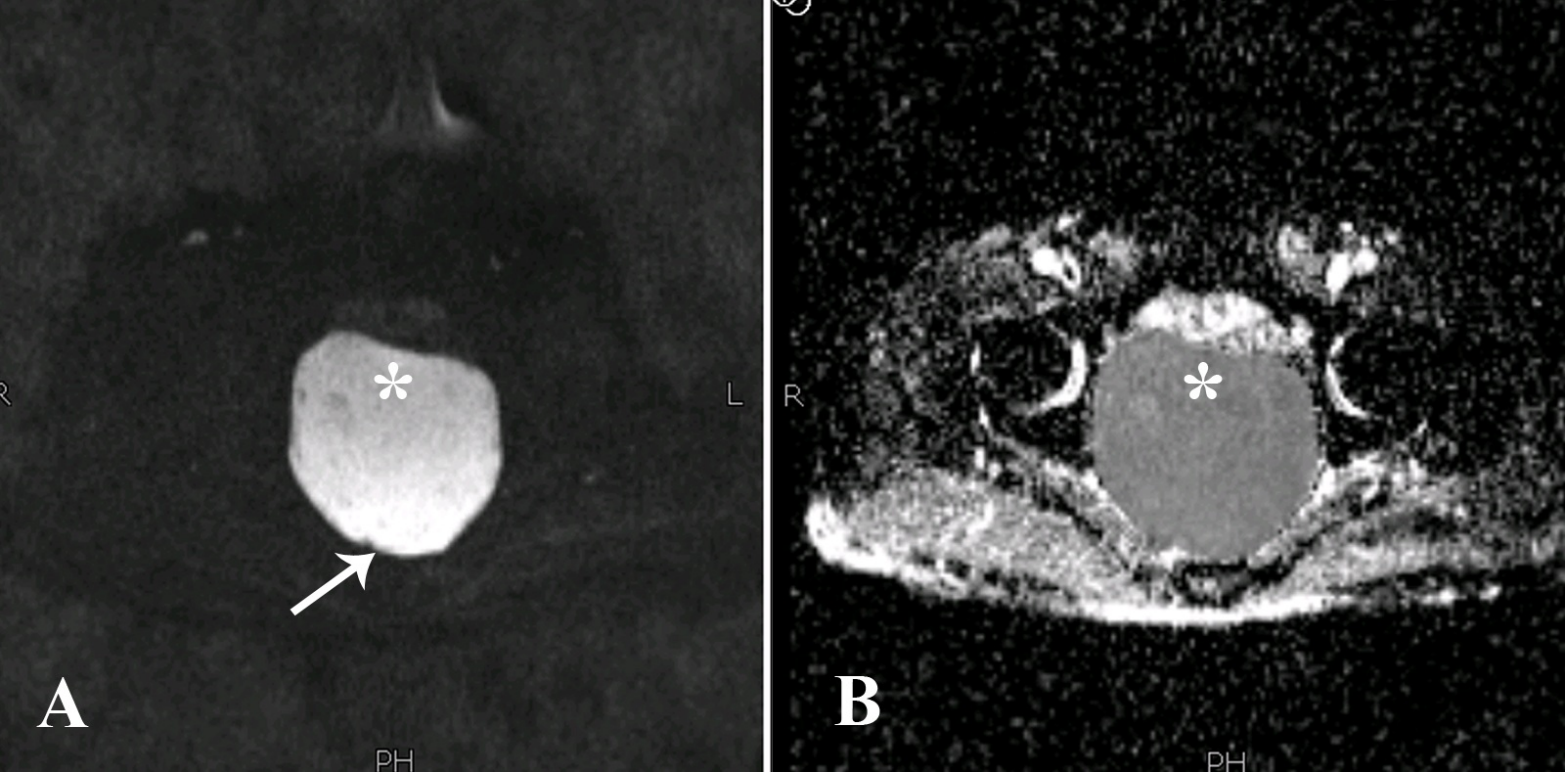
Diffusion weighted sequence (A) and corresponding apparent diffusion coefficient map (B). The lesion (asterisk) was showing diffusion restriction appearing hyperintense on diffusion weighted imaging (A) and showing dropout on the corresponding apparent diffusion coefficient map (B). In addition, there was differential restriction in which posteriorly there were relatively more hyperintense signals on DWI (arrow). DWI: diffusion weighted imaging
